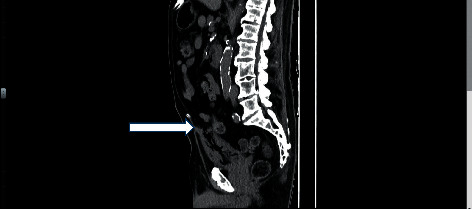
Axial view of the CT scan image demonstrating small bladder perforation and emphysematous cystitis.
